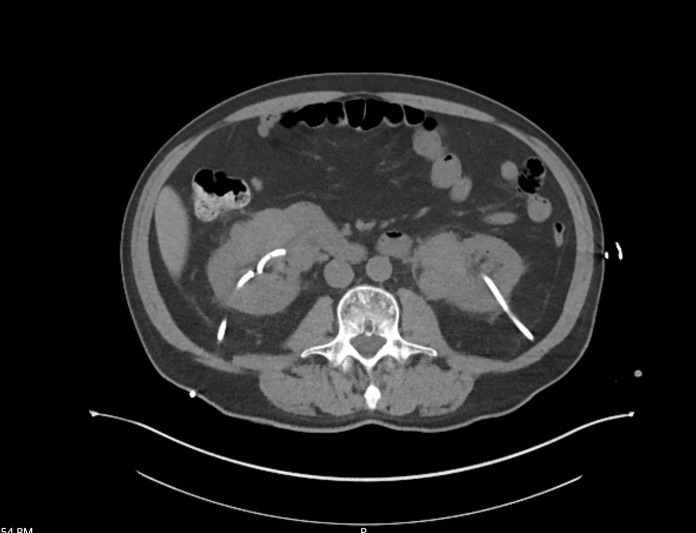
Computed tomography, coronal view in the abdomen demonstrating bilateral kidneys with nephrostomies in place and bulky plasmacytomas at inferomedial border of kidneys bilaterally.
